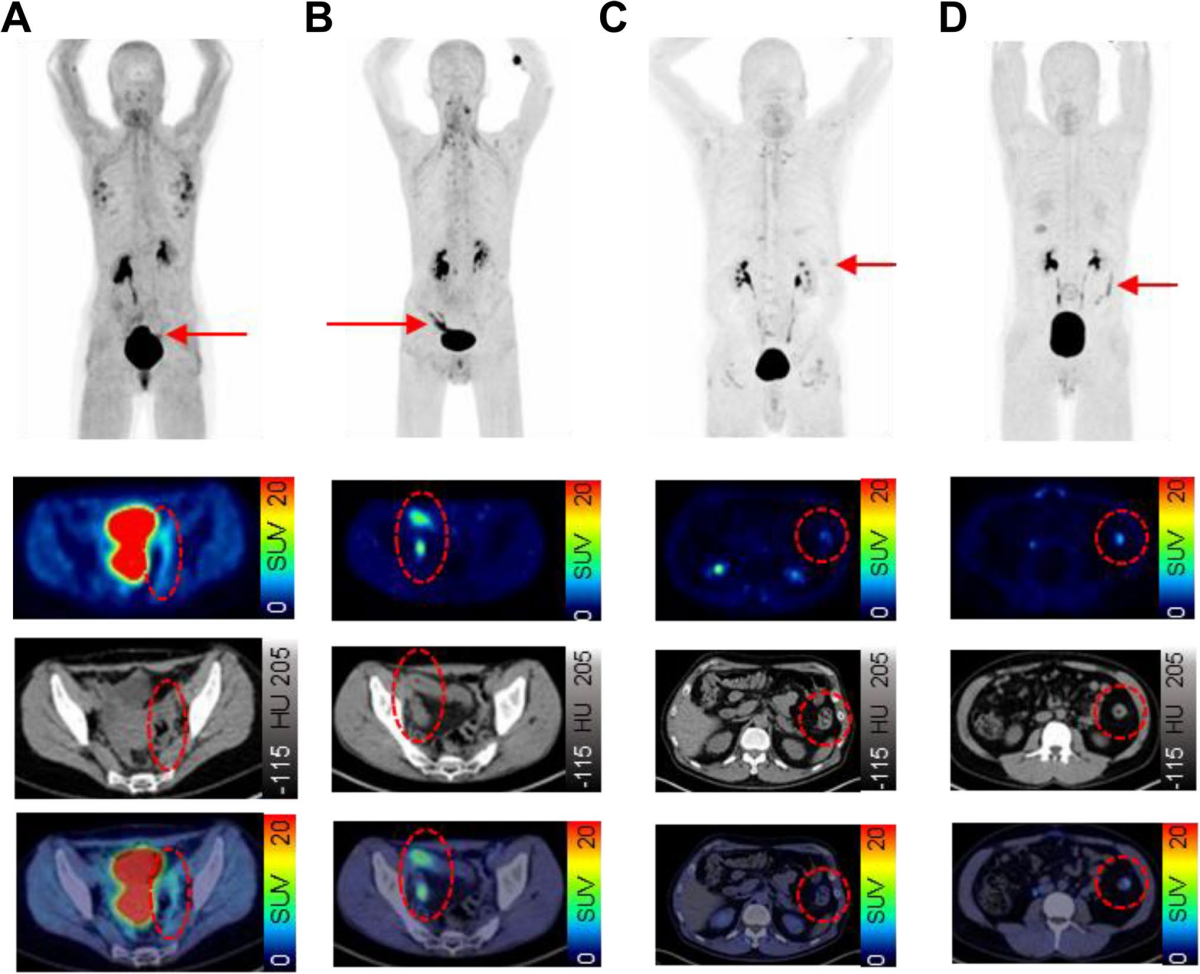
Exemplary cases of inactive and active Crohn´s disease (CD) and ulcerative colitis (UC) of static ^68^Gallium- fibroblast activation protein inhibitor-based radiopharmaceuticals-46-positron emission tomography/computed tomography (^68^Ga-FAPI-46-PET/CT) imaging. For each case, we show maximum intensity projection (MIP) (upper row) and transversal PET, CT and fused images (second, third and fourth row). FAPI-positive inflammatory bowel disease (IBD) lesions are indicated by arrows (MIP) and red circles (transversal images). A CD, inactive disease: 40-year old female with CD in remission. FAPI-positive IBD lesion (SUVmax: 3.3) in sigmoid colon. B CD, active disease: 38-year old male with active CD. FAPI-positive IBD lesion (SUVmax: 8.0) in ileum. C UC, inactive disease: 70-year old male with inactive UC. FAPI-positive IBD lesion (SUVmax: 2.8) in descending colon. D UC, active disease: 30-year old male with active UC. FAPI-positive IBD lesion (SUVmax:5.9) in descending colon
